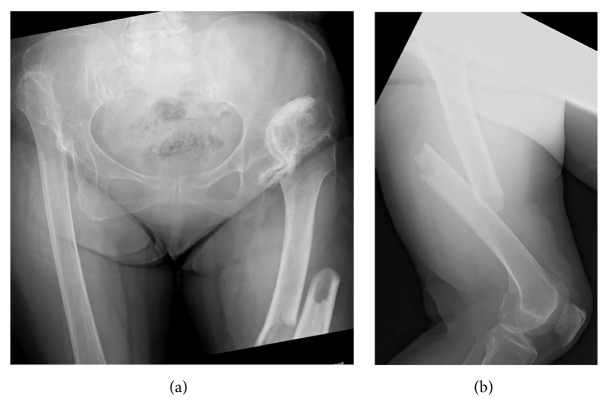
(a) AP and (b) lateral radiographs show a characteristic atypical femoral fracture and severe osteoarthritis of the hip.
